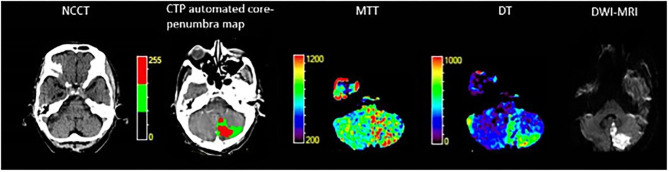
74-year-old male who presented with vertigo, left dysmetria and left sided weakness. NCCT, Non contrast computed tomography; CTP, Computed tomography perfusion; MTT, Mean transit time; DT, Delay time; DWI-MRI, Diffusion weighted imaging—magnetic resonance imaging.
